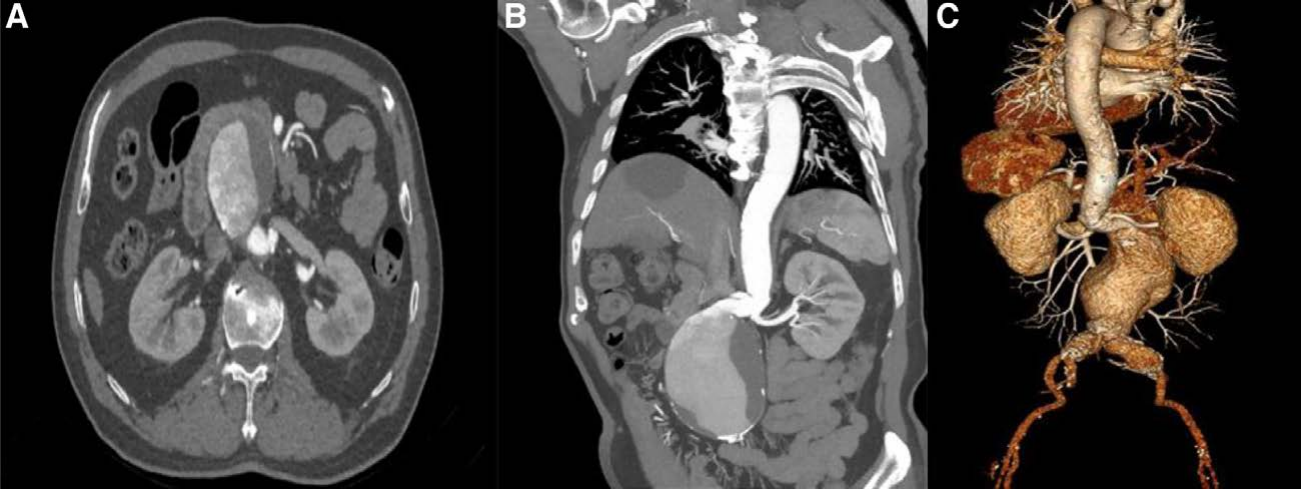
Preoperative computed tomography angiography revealed the infrarenal abdominal aortic aneurysm. A: Axial image of the abdominal cavity; B: Sagittal image; C: 3D reconstruction.
